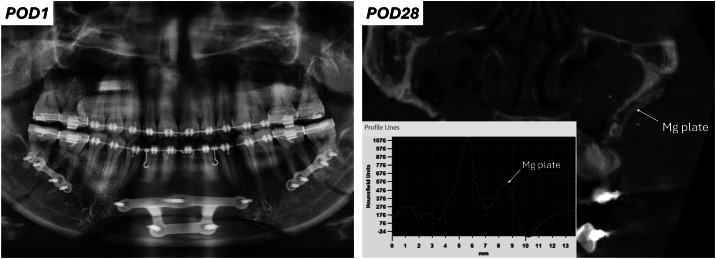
Representative postoperative imaging of magnesium fixation following Le Fort I osteotomy. Panoramic radiograph shows well-positioned maxilla, while magnesium plates and screws are invisible. CBCT confirms visible Mg plate along the lateral maxillary wall, while the radiodensity of Mg is lower than the cortical bone.
